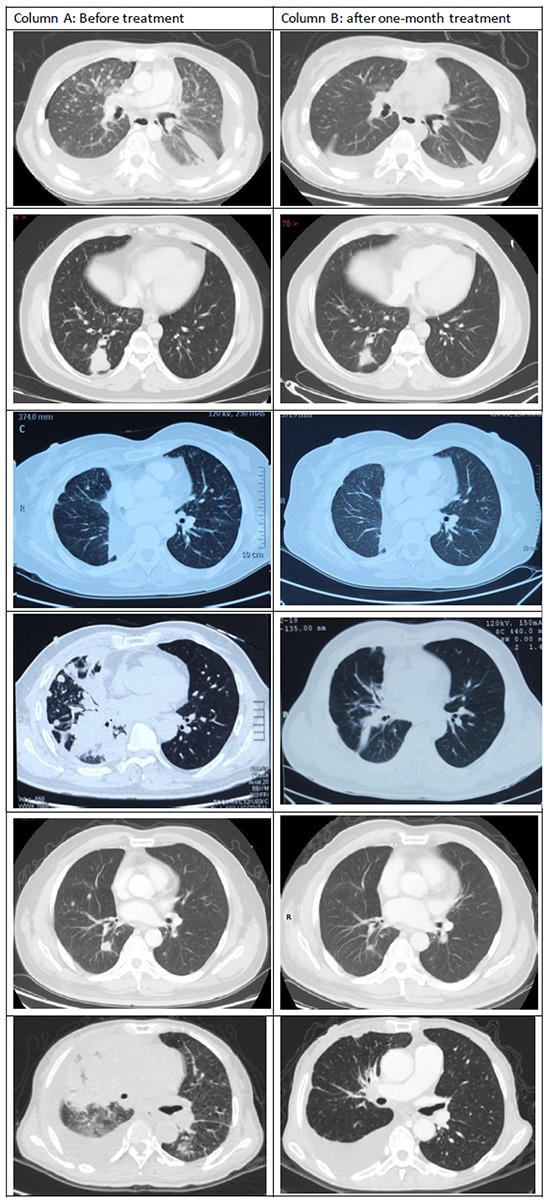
Comparisons of initial computed tomography (CT) images of the thorax with CT images at 1 month after initiation of crizotinib treatment Compared with the initial CT images (Column A), repeated CT imaging at 1 month after the initiation of crizotinib treatment (Column B) showed dramatic shrinking of the cancers in Patients 1, 3, 4, and 5.
